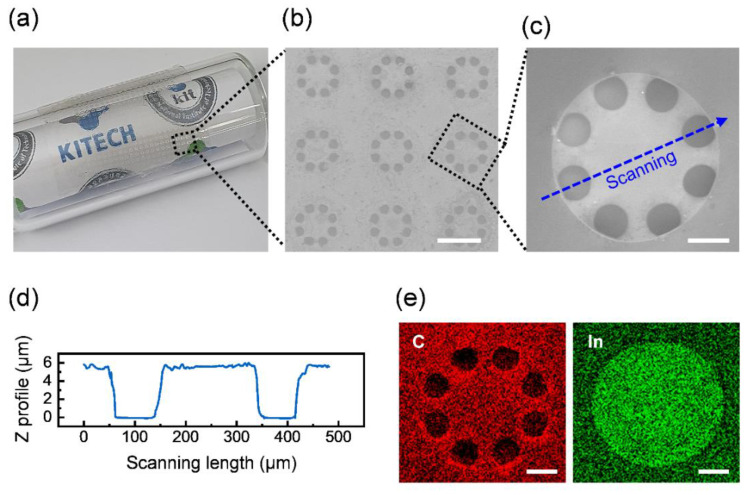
Configuration of the vibration sensor. (a) Photographic showing the transparency and flexibility of the vibration sensor. SEM image of (b) the diaphragm array (scale bar, 400 nm) and (c) a diaphragm structure of the vibration sensor (scale bar, 100 nm). (d) Height profile of cross-sectional view for the suspended diaphragm structure. Scanning route of (d) is shown in the dashed arrow of (c). (e) Carbon and indium elemental mapping data obtained using energy-dispersive X-ray mapping analysis for the same diaphragm in the SEM image (c) (scale bar, 100 nm).
